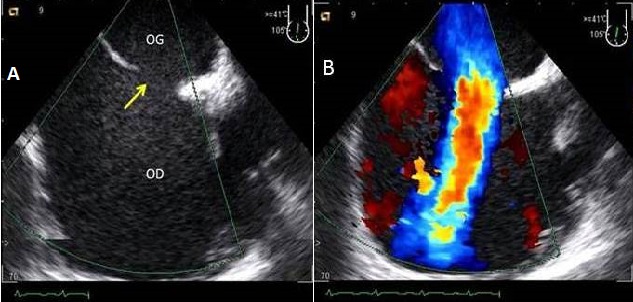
ETO coupe biatriale objectivant une large communication interatriale (flèche) 2D (A) et Doppler couleur montrant le flux de CIA
